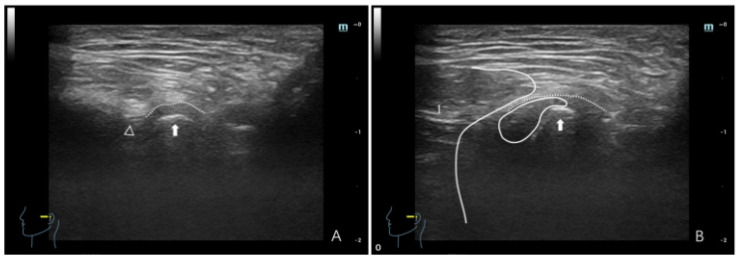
Ultrasonographic image of a TMJ with ADDWoR. (A) Maximum intercuspal position; (B) full opening position. In panel (A), the dotted line denotes the joint capsule, depicted as a hyperechoic (white) line. The white triangle denotes the articular eminence, whereas the white arrow indicates the condyle, where the condyle surface manifests as a hyperechoic line. In panel (B), (1) denotes the masseter, depicted as hypo-to-isoechoic, with white lines delineating the border of the masseter. The dotted line denotes the joint capsule, whereas the white arrow indicates the condyle. The white region between the two denotes the articular disc, which is situated anterior to the condyle.
